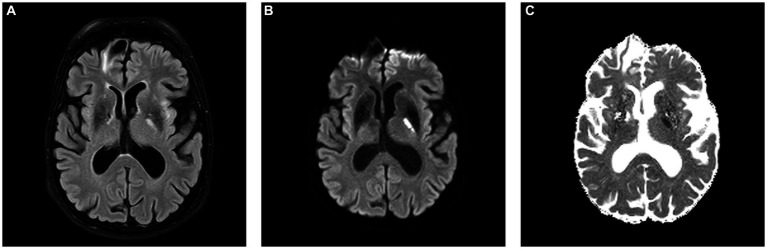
Brain MRI. Acute ischemia of the posterior limb of the left internal capsule. (A) FLAIR transverse image, (B) DWI transverse image, (C) ADC transverse image.
